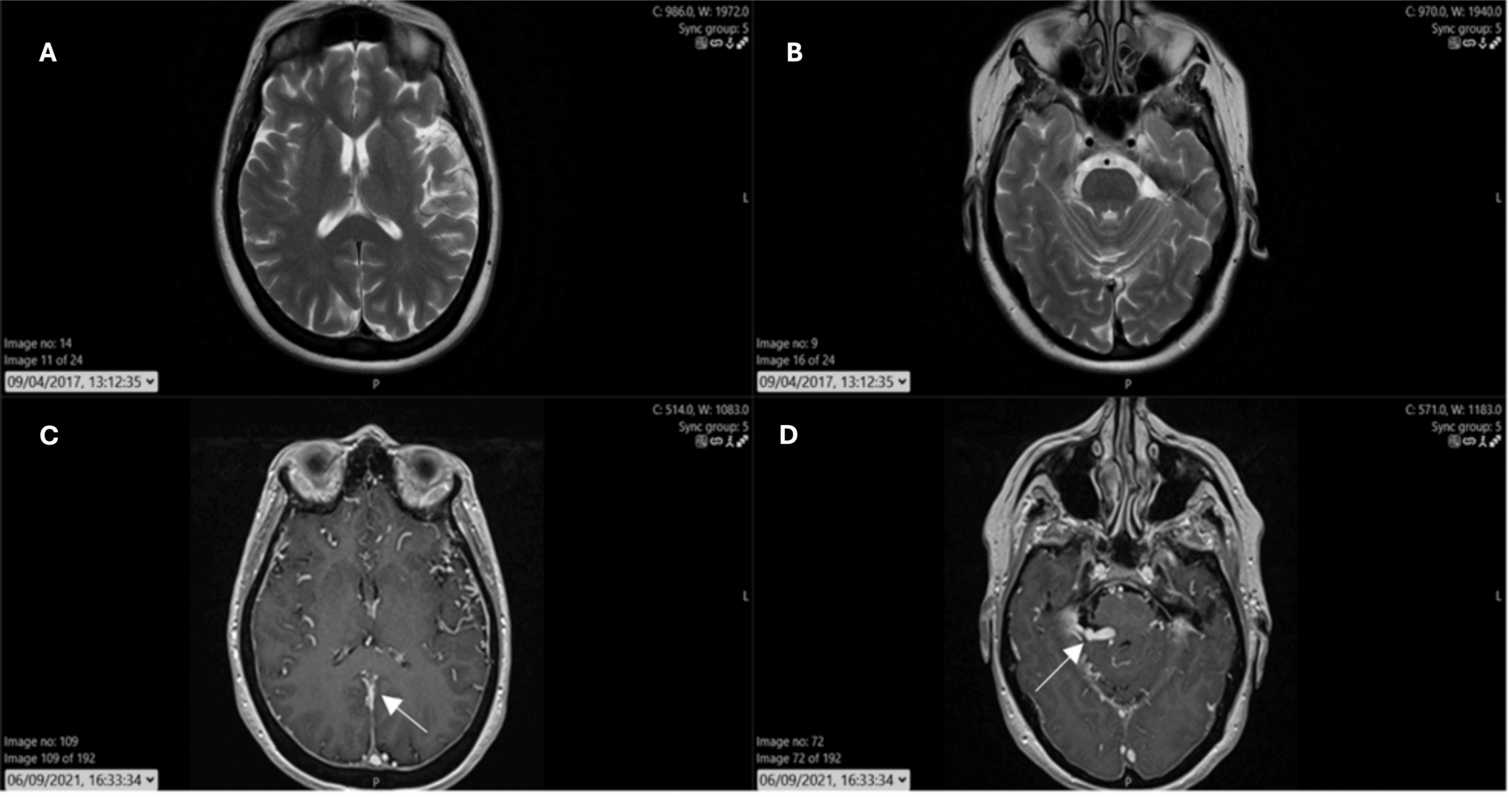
MRI brain. (A) and (B): T2 scan 2017 is grossly normal with no evidence of intracranial hypertension or vascular abnormality (unfortunately, no contrasted T1 available). (C) and (D): T1 with contrast scan 2021, demonstrating global venous engorgement, with dilated vessels supratentorially and infratentorially.
